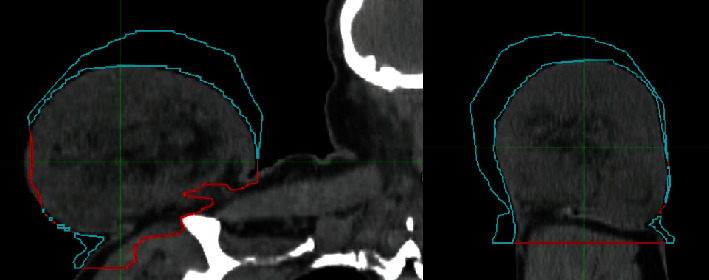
CT of right shoulder postradiotherapy treatment (blue lines indicate tumour volume reduction) in frontal and sagittal planes.
